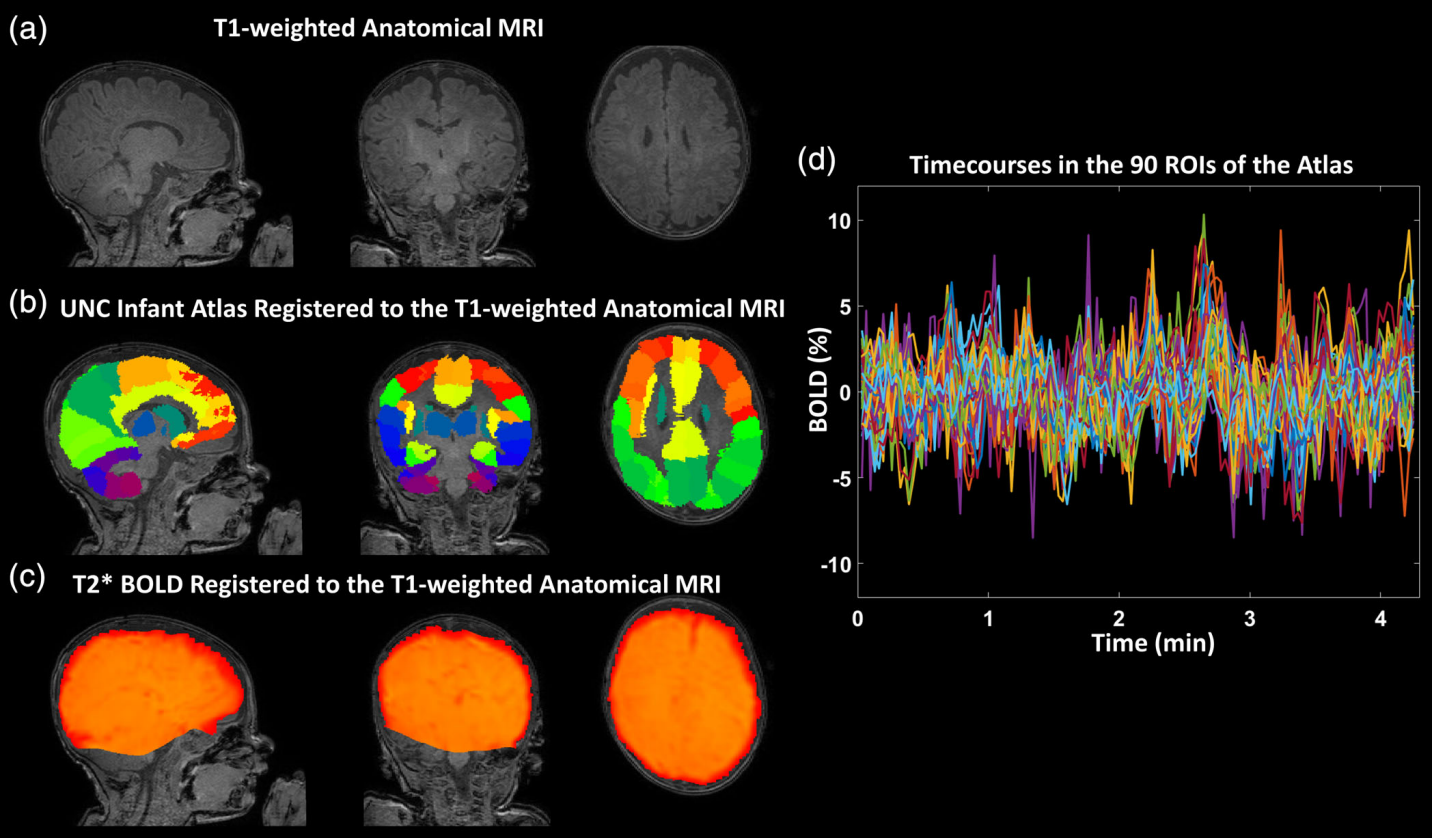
(a) Example of a neonate T1‐weighted anatomical MRI. (b) UNC Infant Atlas registered to the T1‐weighted MRI. (c) Motion‐corrected functional BOLD volume registered to the T1‐weighted MRI. (d) 90 preprocessed BOLD signals extracted for the same infant from the ROIs defined in the atlas by transforming the anatomy‐registered atlas into the native BOLD space
